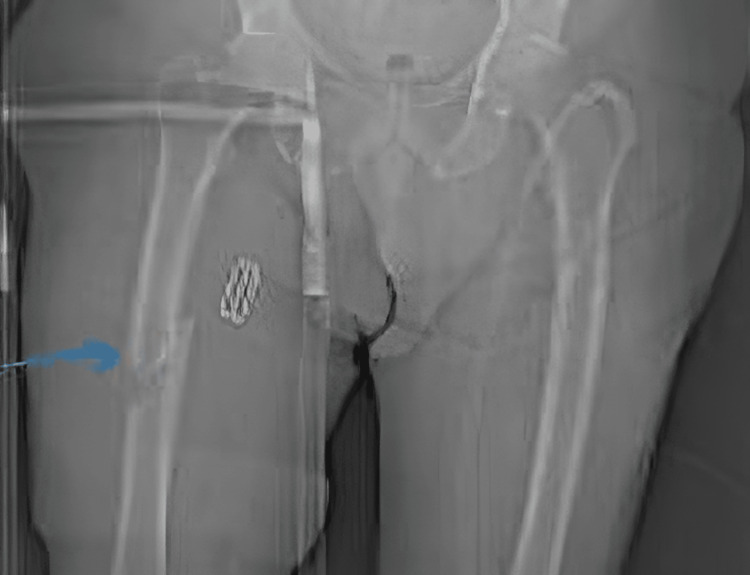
CT of the right thigh without contrast showing a pathologic fracture through a lytic lesion of the mid-femoral shaft Arrows indicate the cortical destruction and fracture line. CT: computed tomography
